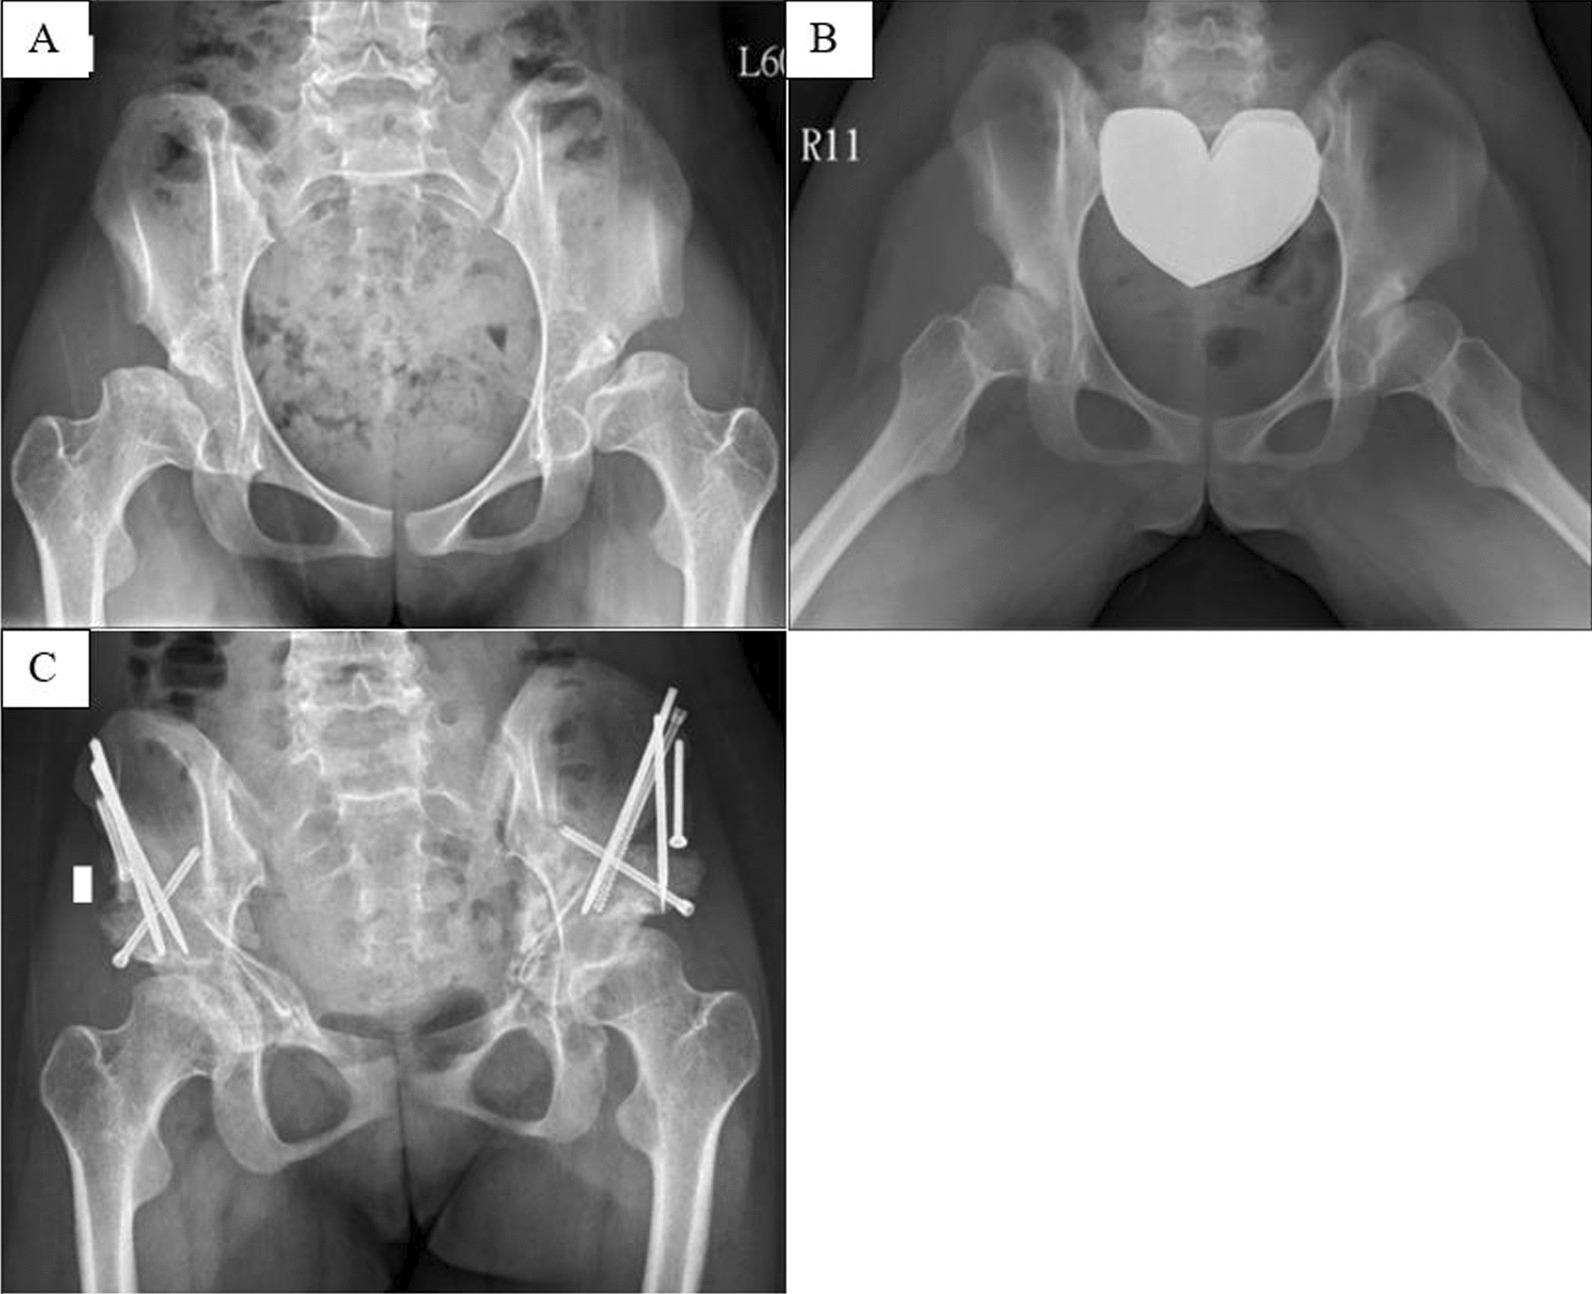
The comparisons between preoperative and postoperative outcomes in the anterior–posterior pelvic radiograph
